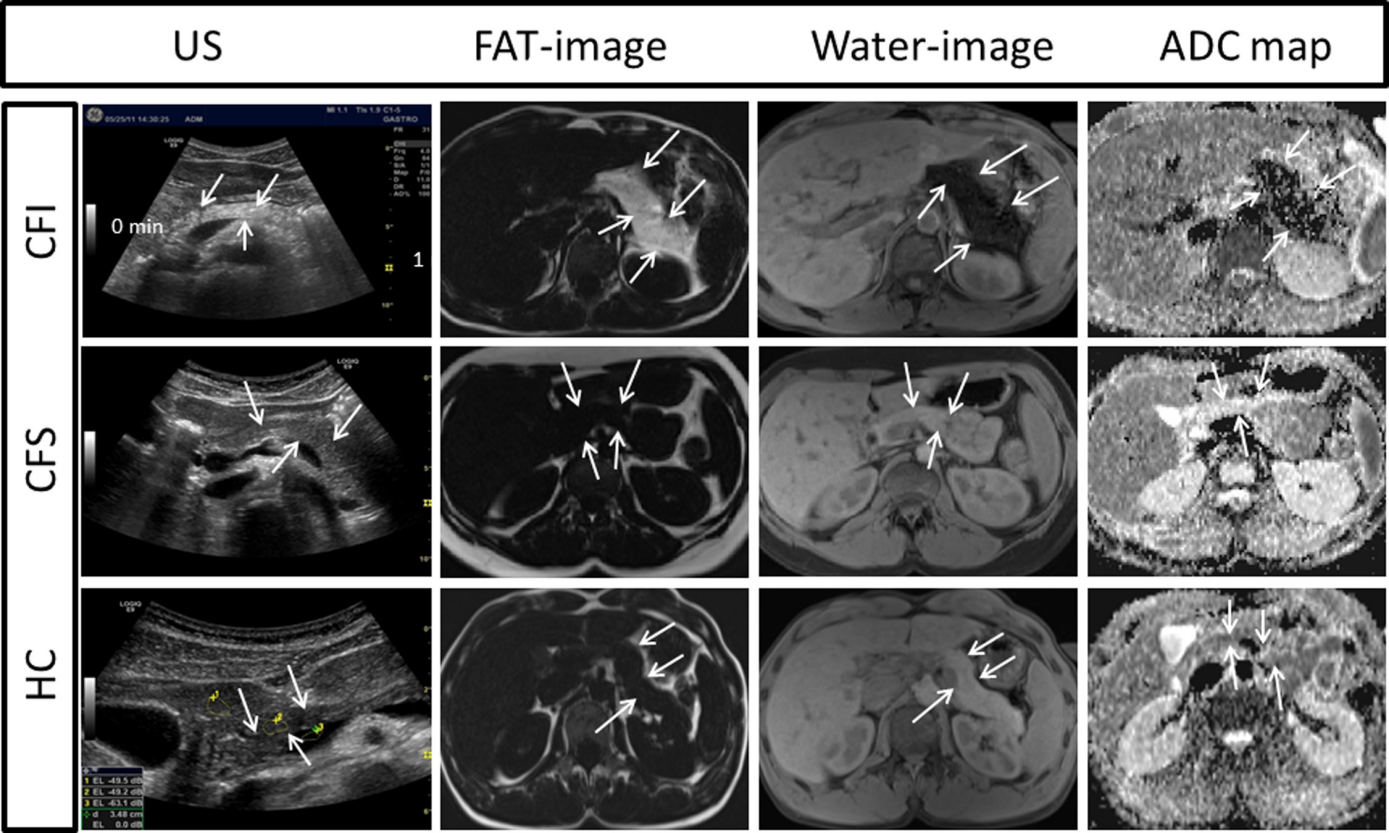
US (left column), Dixon MRI (middle columns) and ADC maps (DWI) (right column) images of the pancreas in patients with CFI (upper row); CFS (middle row) and HC (lower row). The CFI patients typically exhibited pancreatic hyperechogenicity (US), increased pancreatic fat content demonstrated by hyperintensity on fat image and hypointensity on the corresponding water image (Dixon MRI) and restricted diffusion with pancreatic hypointensity on the ADC map (upper row), whereas none of the CFS patients or HCs had signs of increased pancreatic fat content or restricted diffusion (middle and lower row). The boundaries of the pancreas are indicated with arrows and the selected ROIs in the liver, pancreas and vessel of US are marked in the HC subject. ADC: apparent diffusion coefficient, CFI: Pancreas insufficient cystic fibrosis, CFS: Pancreas sufficient cystic fibrosis, DWI: Diffusion weighted imaging, HC: Healthy control, US: ultrasound.
